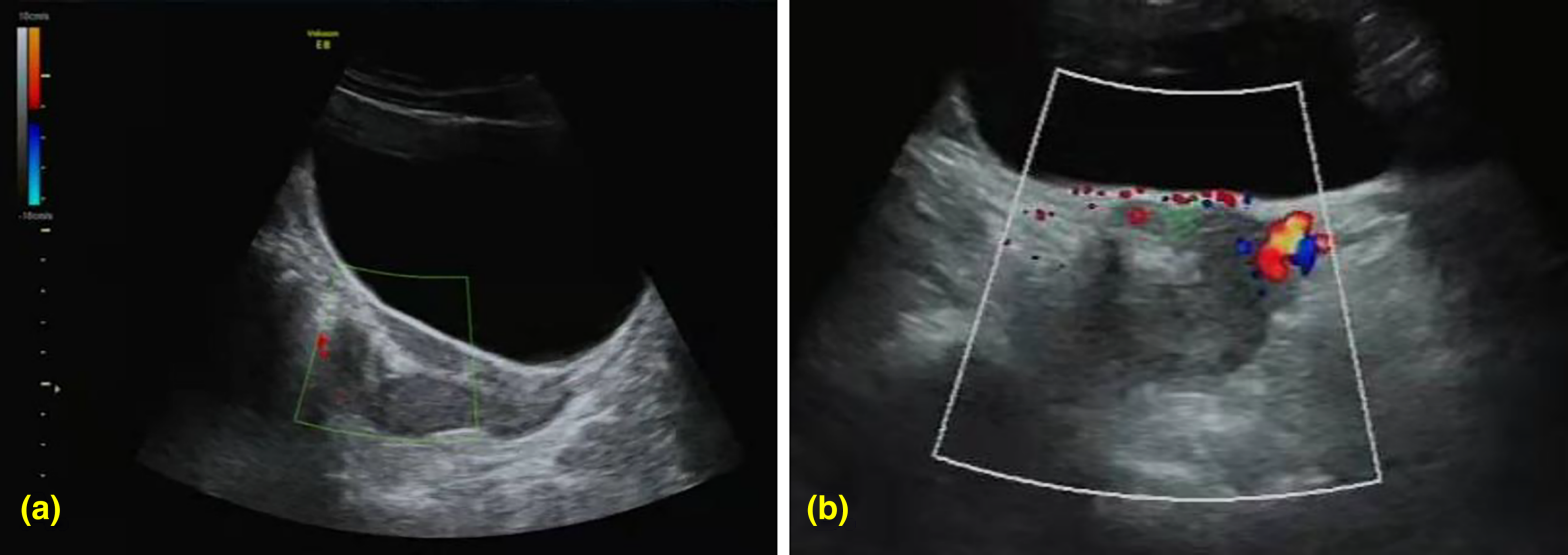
Ultrasound image shows the presence of a hyperechogenic structure in the anterior wall of the uterus (a, b).
